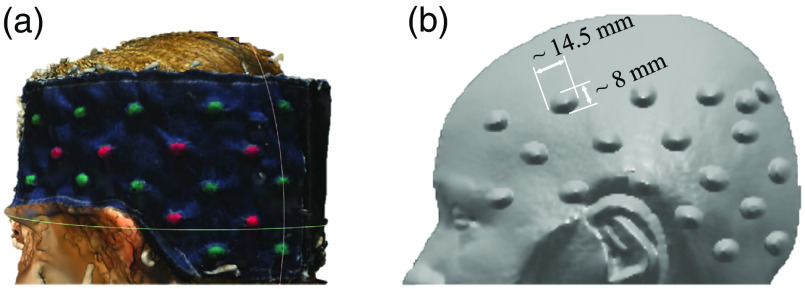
Reconstructed head images with optode-set by (a) POR and (b) MRI registration methods.
